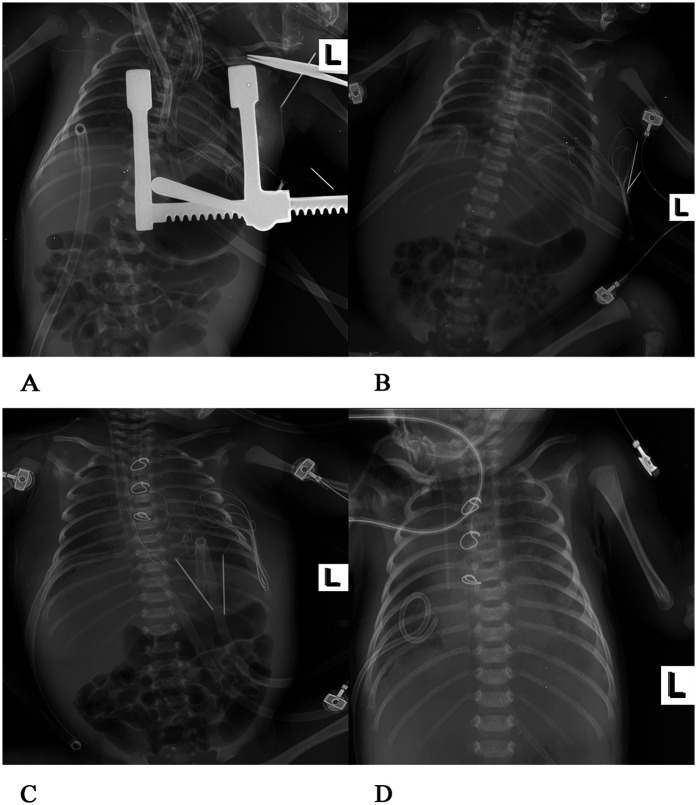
Postoperative chest x-rays at various stages (A) 3 h after operation of congenital heart disease, blood in both lungs was normal, there was no consolidation in both lungs, and the shape and size of the heart were normal. (B) On the 6th day after surgery, there was a light shadow in both lungs, a small amount of exudation in both lungs, and soft tissue swelling in both thoracic and abdominal walls, and the tip of the tracheal intubation was flat at the upper margin of the second thoracic vertebra. (C) On the 8th day after surgery, the transmittance of both lungs was reduced, and flaky-like blurred shadows were visible. A strip of high transmittance was visible in the upper right lung with the edge of compressed lung tissue, a right pneumothorax, a heart of normal shape and size, and the tracheal intubation tip was flat at the third thoracic vertebra. (D) On the 23rd day after surgery, a large flake high-density shadow was seen in both lungs, the metal ring was intact, no fracture was observed, both lungs exuded, right upper lung atelectasis, and the heart shape was normal.
